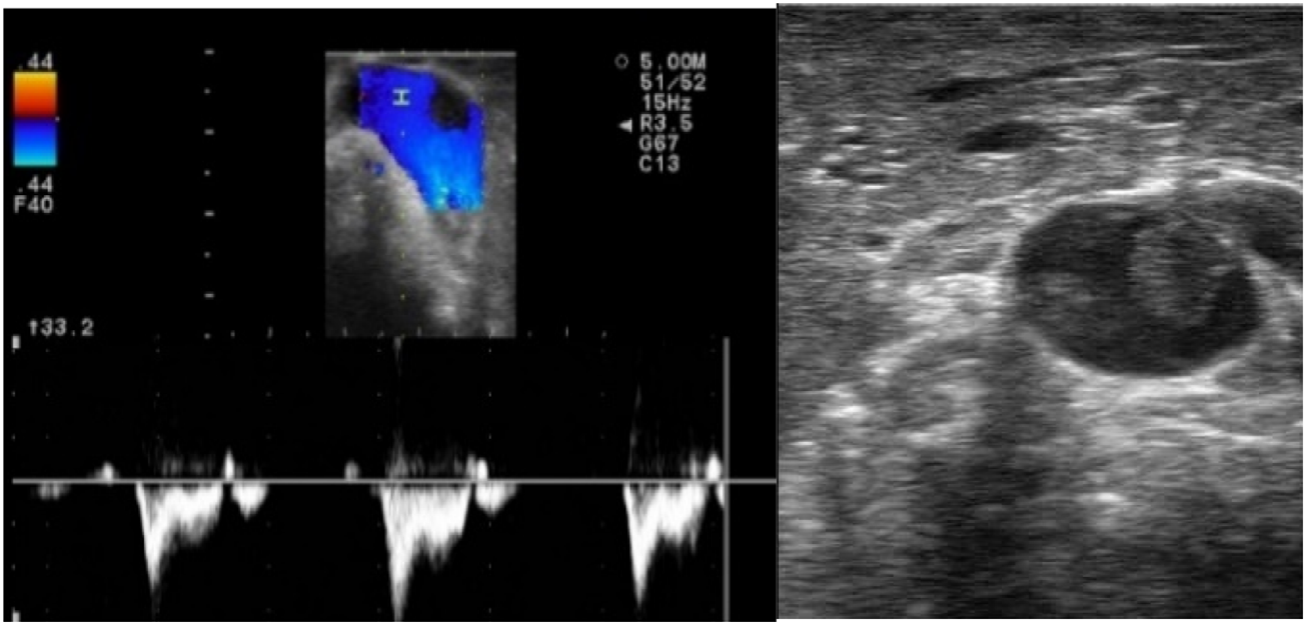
Intraoperative ultrasonography of pulmonary embolism. (a) Doppler image. The visible filling defect is a thrombus. (b) A clot can be seen in the blood vessel.
